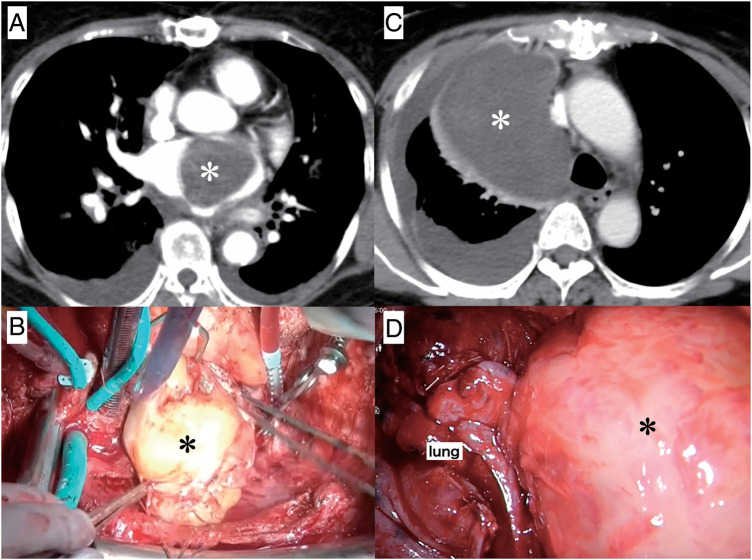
Follow-up tomography results. The CT radiographs acquired 2 years after the first surgery (A) revealed tumor (asterisk) recurrence. The operative photograph taken during redo surgery (B) shows a myxomatous tumor (asterisk), similar to the first one, being removed from the left atrium. The CT radiograph acquired 5 months after the last surgery (C) showed a tumor (asterisk) in the right pleural cavity. The thoracoscopic image (D) showed a large tumor (asterisk) adhering to the lung. CT, computed tomography; IVC, inferior vena cava; SVC, superior vena cava
